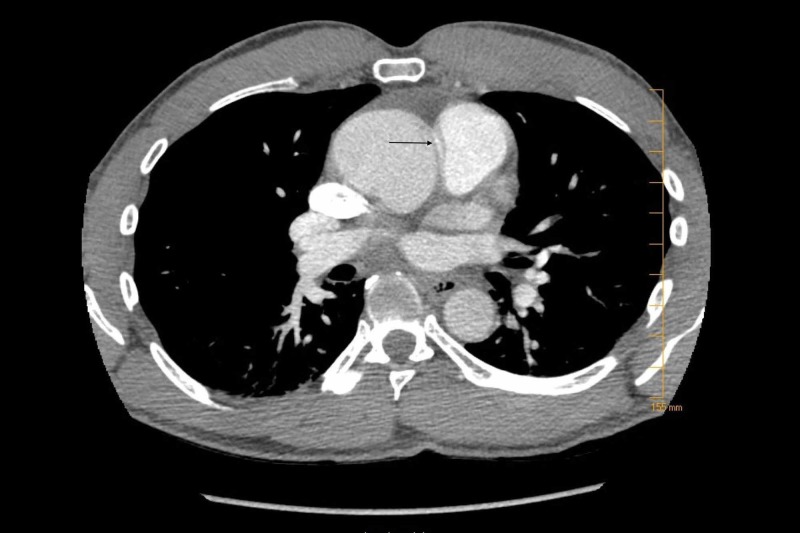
Computed tomography (CT) of the chest (axial view); arrow showing aberrant origin right coronary artery
